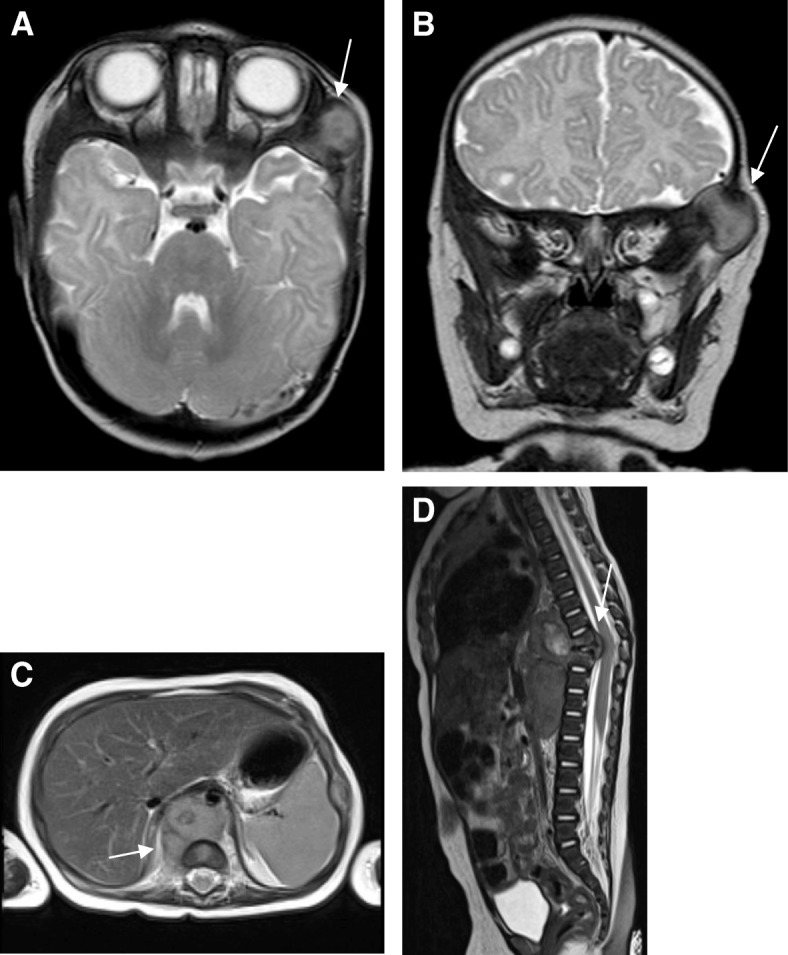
Magnetic resonance imaging. a head transversal and b head coronal T2 TSE: intraosseous soft tissue mass in the left sphenoid bone. c trunk axial T2 BLADE: prevertebral mass with elevation of diaphragm and thoracal and abdominal aorta. d spine sagittal T2 TSE: vertebra plana Th9, adjacent Th8 and Th10 wedge-shaped, thoracal gibbus, compromise of spinal cord by intraspinal part of Th9
